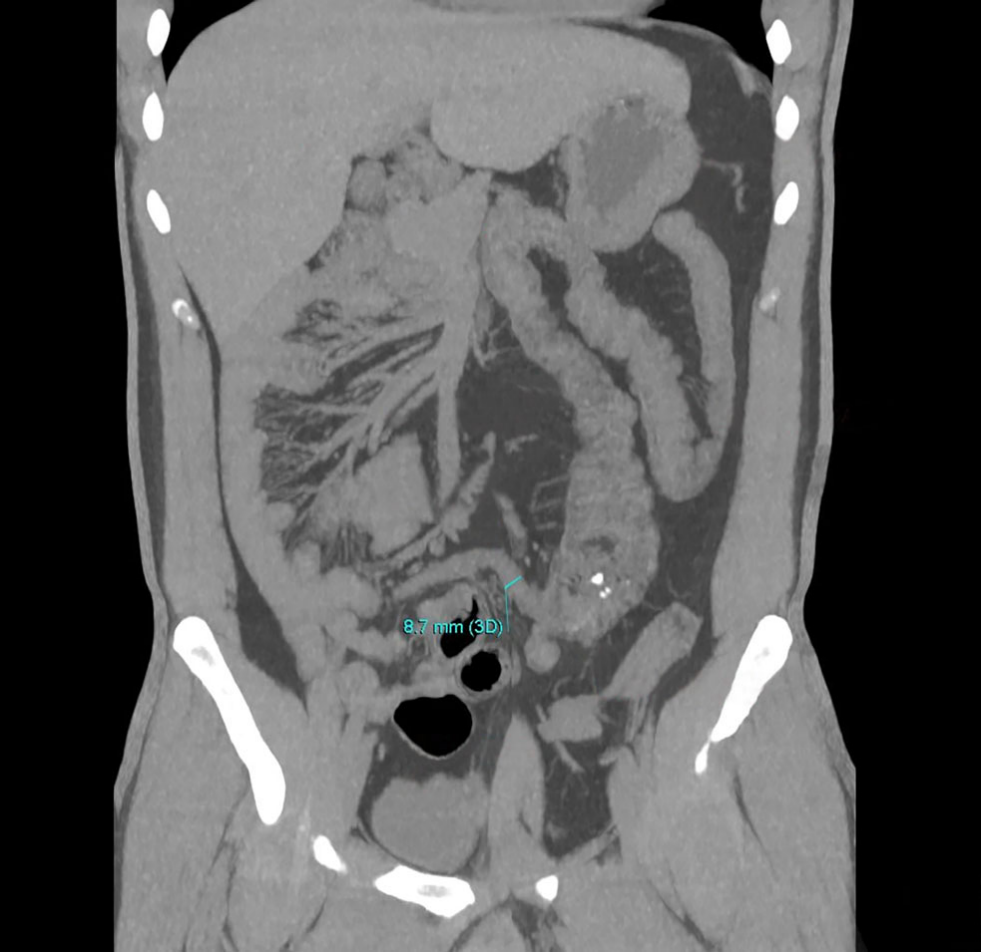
Coronal maximum intensity projection reconstruction computed tomography image showed dilated appendix in the left mid‐abdomen with congenital gastrointestinal malrotation. The appendix was located at the umbilicus with a diameter of about 8.5–10 mm, containing fluid inside and surrounding fat infiltration.
